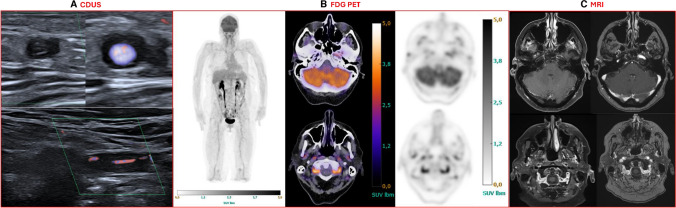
Example images of a GCA patient. A. CDUS images of the right temporal artery (transverse view, upper images) and left vertebral artery (longitudinal view, lower image). A halo sign may be observed in both arteries. B. FDG PET images with from left to right maximum intensity projection (MIP), fused PET/CT images of the head, and native PET images of the head. MIP image shows increased uptake in the common carotid arteries (grade 3 against liver) and the images of the head show increased uptake in the common superficial temporal and occipital arteries (grade 1 against background, upper images) and the vertebral and external carotid arteries (grade 2 against background, lower images). Grade 2 and 3 were considered positive for GCA. C. MRI images of the head with contrast enhanced T1-weighted MRI on the left and time-of-flight MRA on the right. Vessel wall thickening was observed in this patient in the common superficial temporal arteries and occipital arteries (both grade 3), most clearly visible here on the left. The lower image shows vessel wall thickening of the vertebral arteries (grade 2)
